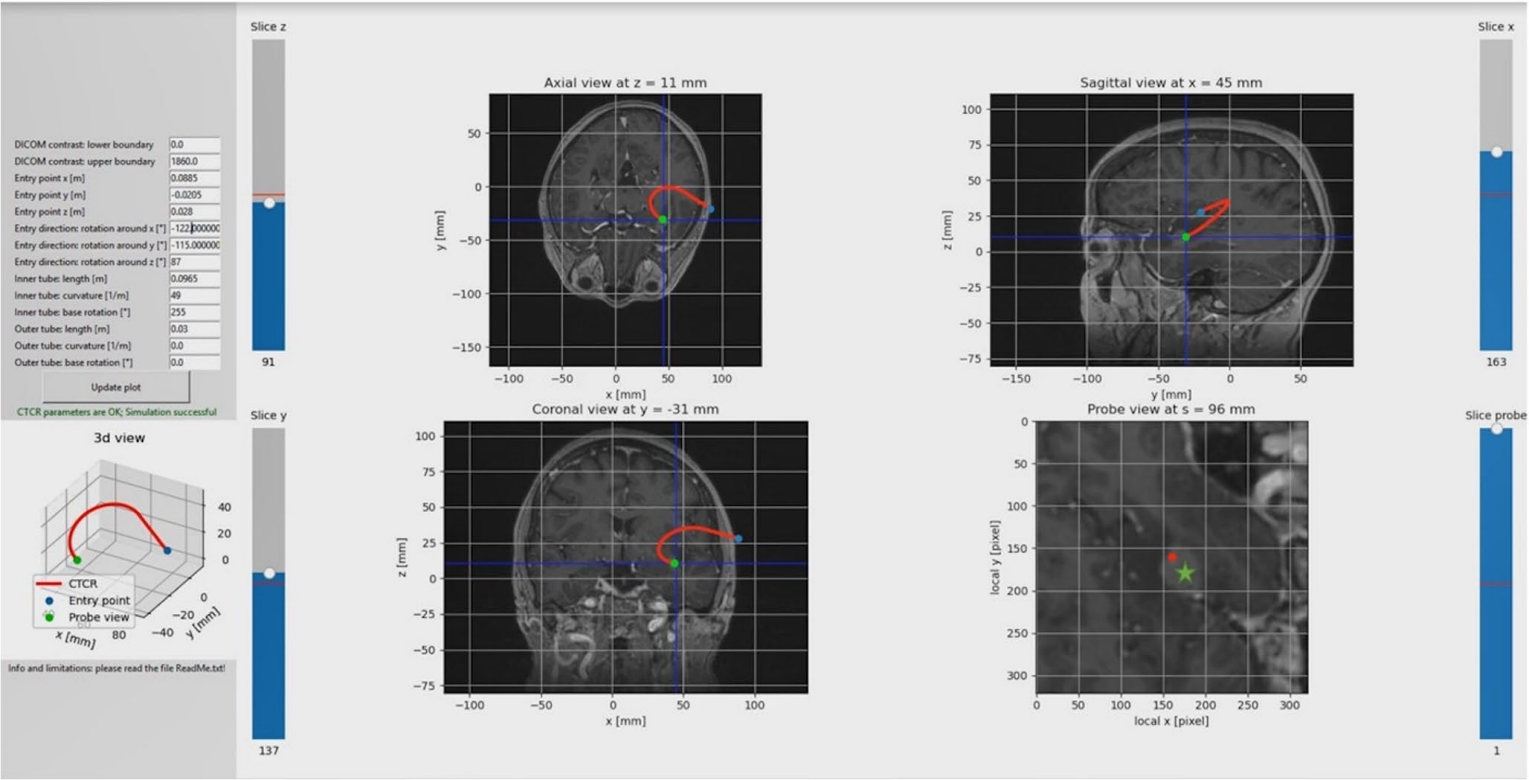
Planning of curved path on MRI-data of a patient with a lesion located in the insular region. Path planning of a curved trajectory. A lesion located at the insular region with weak contrast enhancement (green asterisk) was targeted. Path planning with axial, sagittal and coronar view was difficult. Potential risk factors for complications such as arteries and sulci could be best assessed with the‚ probe’s eye view‘
